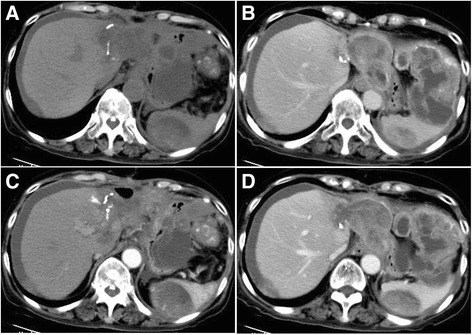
Abdominal CT imaging 47 days after surgical excision. a, d The outer area of the left hepatic lobe is absent. Extensive massive, nodal-like shadows can be found in the liver, spleen, peritoneum, omentum majus, and mesentery. b, c Heterogeneous enhancement is noted. There is no clear boundary between the lesion, the incisal edge of the left hepatic lobe, and the gastric wall. A low-density filling defect can be observed in the main portal vein. A flake-like shadow of liquid density is observed on the surface of the liver and in the inter-intestinal space
